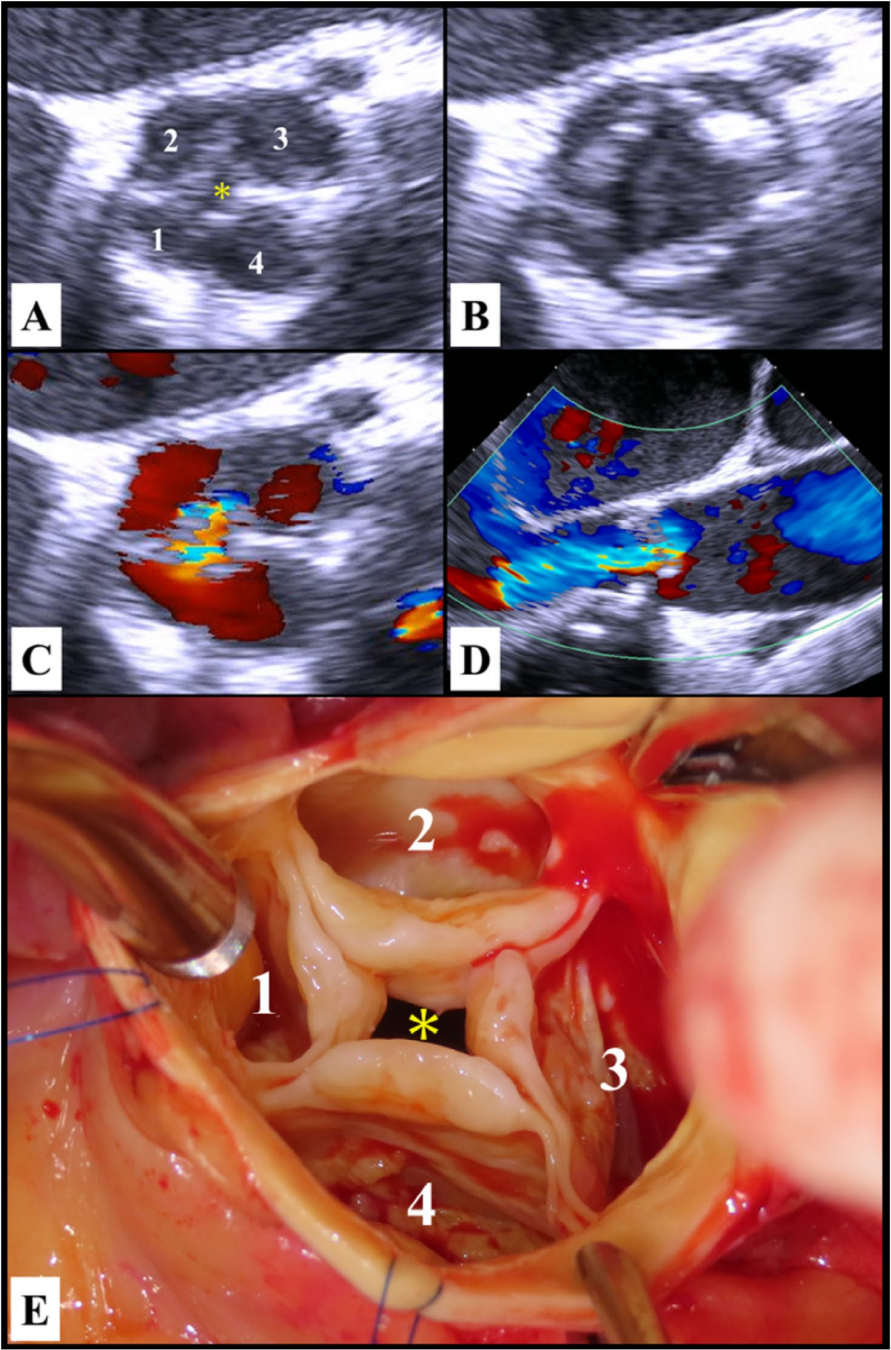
Preoperative transesophageal echocardiography showed four leaflets in the short‐axis view of the aortic valve (A, diastolic phase; B, systolic phase). Severe central aortic regurgitation (C, short‐axis view; D, long‐axis view) resulted from a small diastolic coaptation defect (A and E, asterisk). Intraoperatively, the surgeon's view revealed a quadricuspid aortic valve (E). The four cusps were of equal size and the accessory cusp was located between the right and left cusps (A and E, 1: Right‐coronary cusp, 2: Non‐coronary cusp, 3: Left coronary cusp, 4: Accessory cusp).
